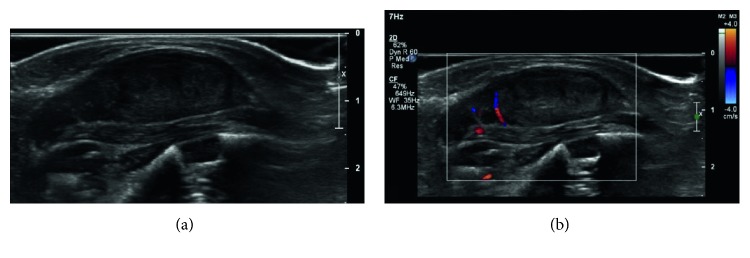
Grayscale (a) and color Doppler (b) sonographic imaging demonstrates a fusiform, heterogeneously hypoechoic mass in the posterior left neck, adjacent to the paraspinal muscle, without significant increased vascularity.
